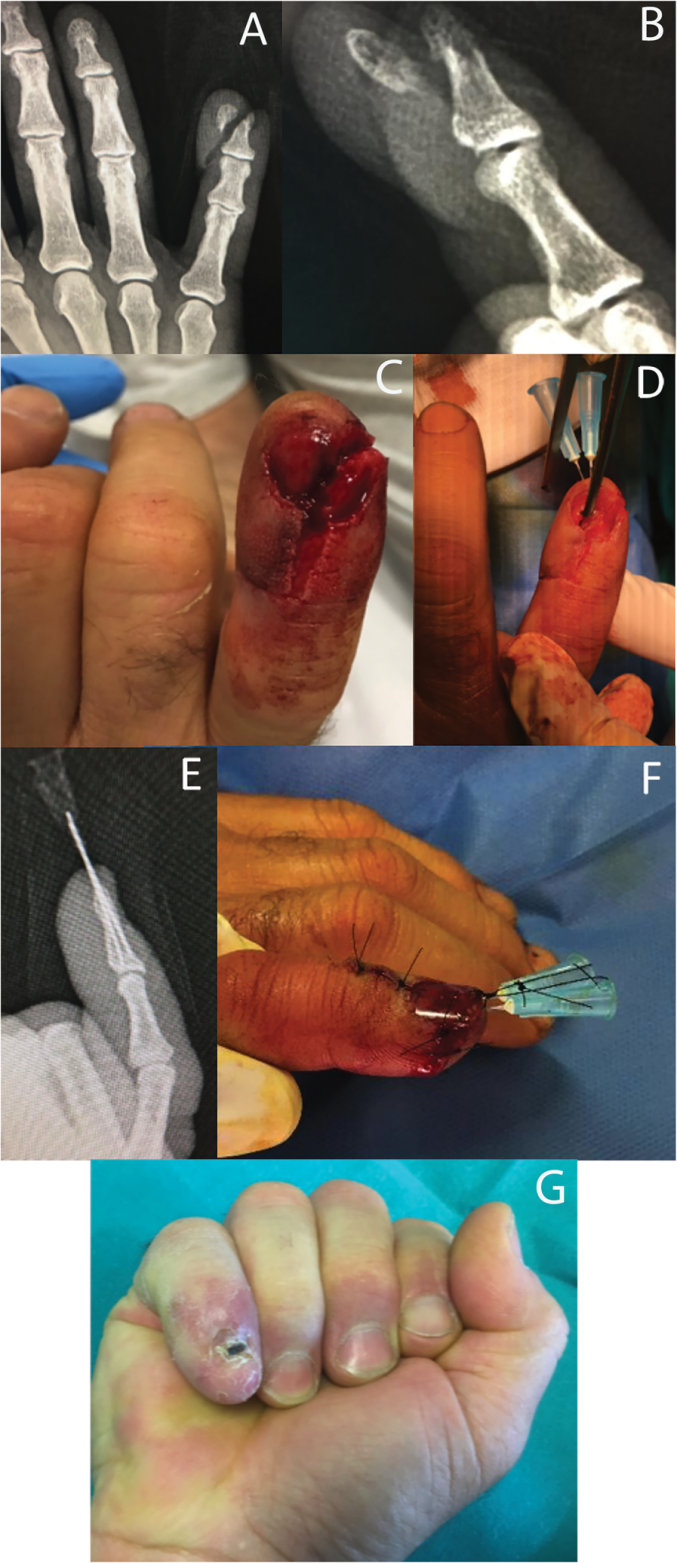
Male patient, 44 years old, with open injury of the distal phalanx and tuft fracture of the little finger (a,b,c). Clinical presentation after fixation of bone fragments with the hand crossed 23-G needles. The nail bed was also restored and a silicon lamina was used to protect it (d,f). X-rays performed 30 postoperatively (e), and clinical outcome after the removal of fixation devices (g)
